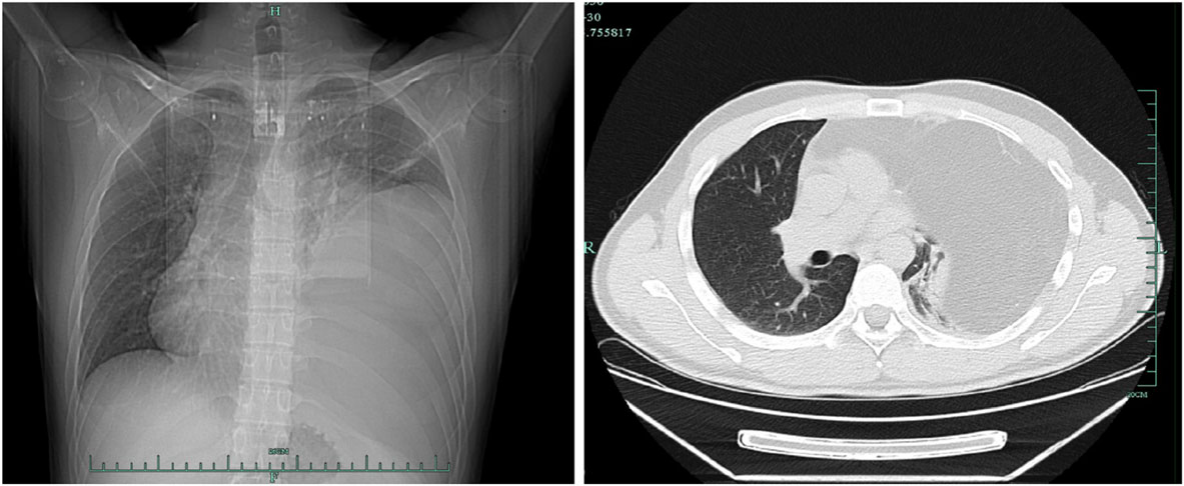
Chest CT scan revealed a huge mass with fat density in the anterior superior mediastinum and left lung field, the inferior lobe of left lung was compressed, trachea and heart shifted to the right side
